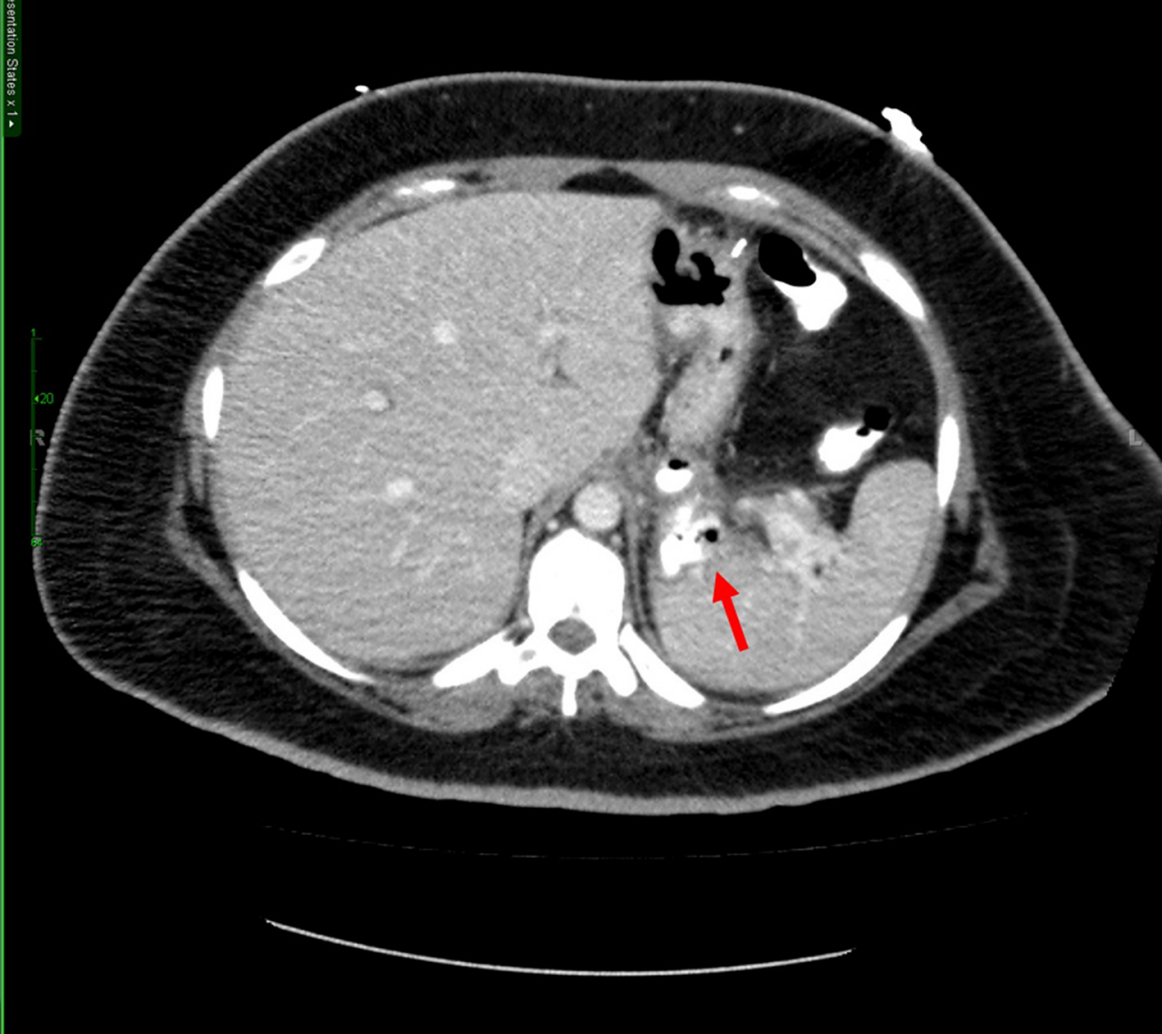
CT scan with contrast confirming leak, late phase. Arrow indicates extravasated extraluminal contrast and air representing leak on CT.
